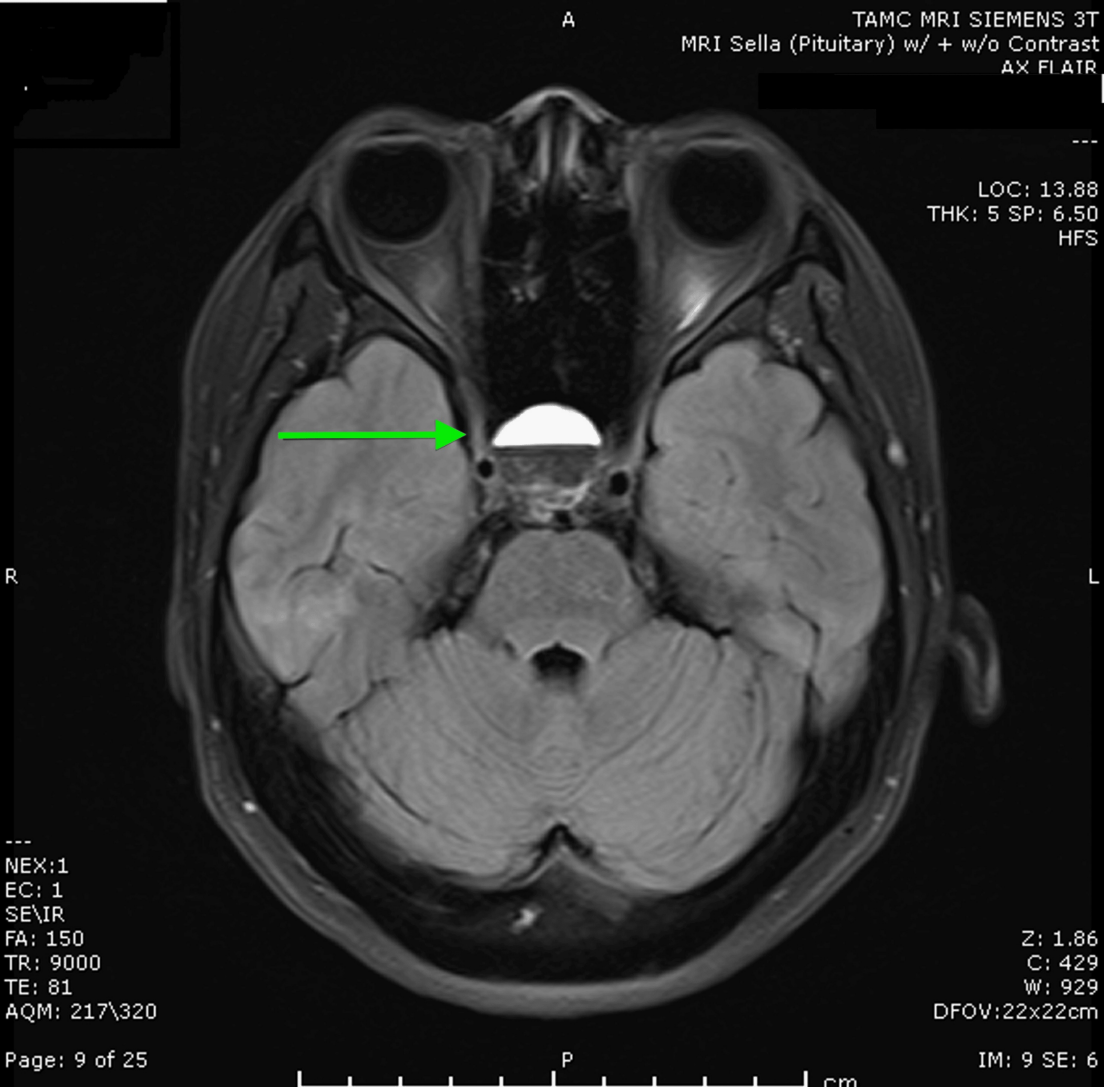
Axial FLAIR MRI image of the pituitary gland showing a hyperintense lesion within the pituitary macroadenoma, consistent with internal hemorrhage. FLAIR: fluid-attenuated inversion recovery
